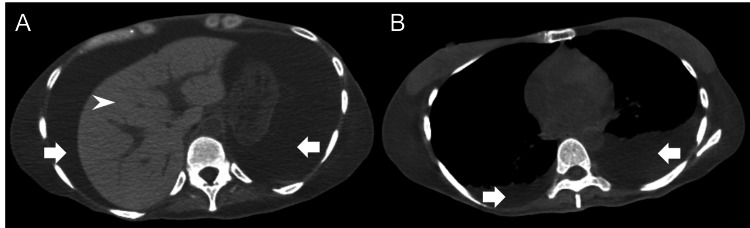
Simple computed tomography (CT) (A) High-density area (73 Hounsfield Units, HU) of the liver parenchyma (white arrowhead), with no gross liver mass visible. (A, B) Thoracoabdominal fluid (white arrow). Chronic inflammatory disease causing hyperferritinemia was not identified.
